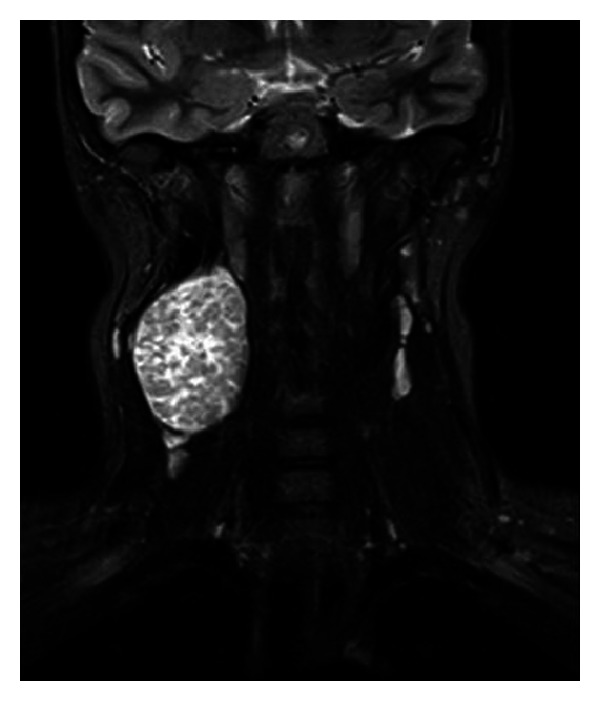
MRI head and neck showing a 3.9 × 3.8 × 6.1 cm lesion with a heterogeneous enhancement pattern and cervical lymph nodes within normal limits.
